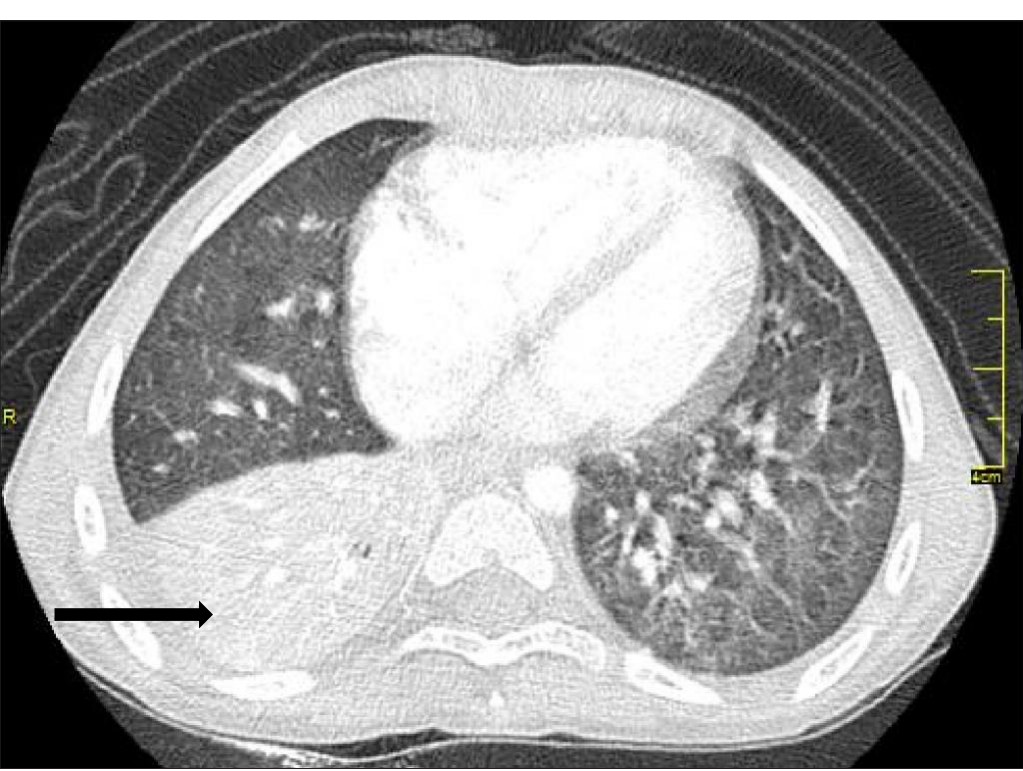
Chest computed tomography scan showing a right lower-lobe
